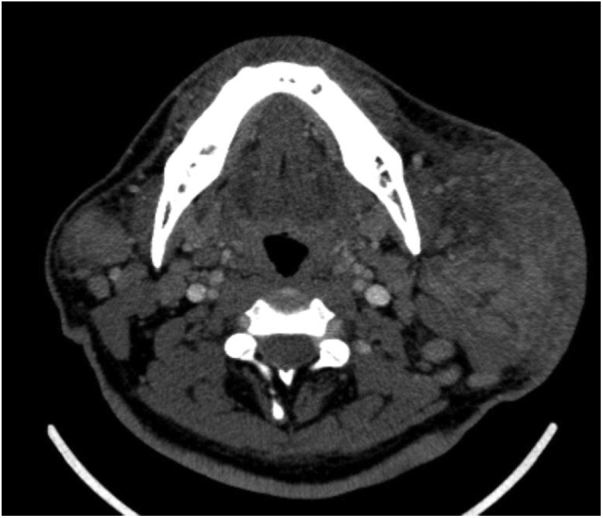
Computed tomography of the neck (axial view) showing extensive fat streakiness of overlying subcutaneous tissue plane with skin involvement over the right. There is no focal enhancing collection seen within the glands.
